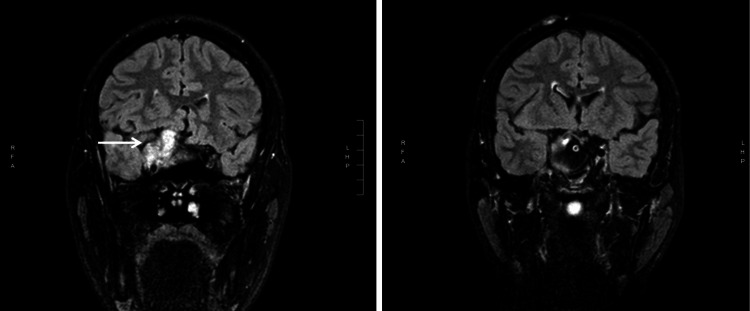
Brain MRI: T2-weighted coronal FLAIR pre-surgery (left image) and post-surgery (right image) FLAIR: Fluid-attenuated inversion recovery
